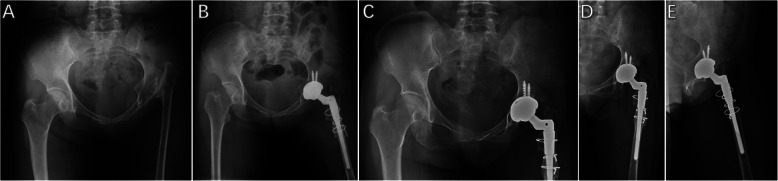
Radiographs of a 50-year-old female patient with high hip dislocation secondary to childhood pyogenic infection that had a quiescent period of 46 years between infection and left THA. a Preoperative AP pelvic radiograph. b Immediately postoperative AP pelvic radiograph after left THA with subtrochanteric shortening osteotomy. c Postoperative AP pelvic radiograph 6 years after THA. d Postoperative AP and e oblique hip radiographs 6 years after THA
